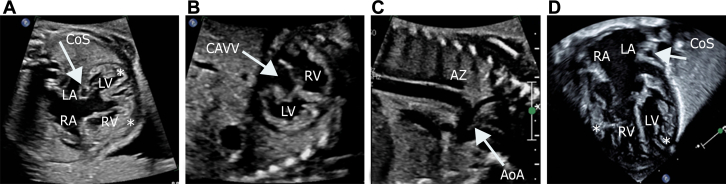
Fetal and Postnatal Transthoracic Echocardiogram Fetal echocardiogram demonstrating (A) 4-chamber view, (B) short-axis view of the ventricles, and (C) sagittal view of the aortic arch. (D) Postnatal 4-chamber echocardiographic view demonstrating the CAVV and ventricular noncompaction. Asterisks indicate noncompaction. AoA = aortic arch; AZ = azygos vein; CAVV = common atrioventricular valve; CoS = coronary sinus; LA = left atrium; LV = left ventricle; RA = right atrium; RV = right ventricle.
